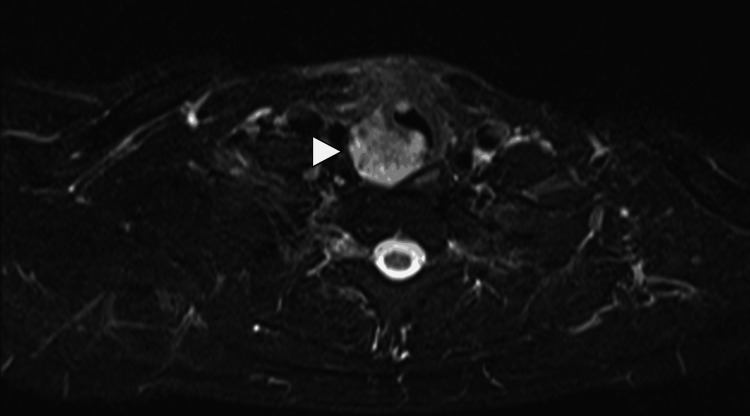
MRI neck with contrast The short Tau inversion recovery magnetic resonance imaging (STIR MRI) image shows a hyperintense contrast-enhancing soft tissue lesion at the right upper para and retro tracheal area extending slightly to the left side.
